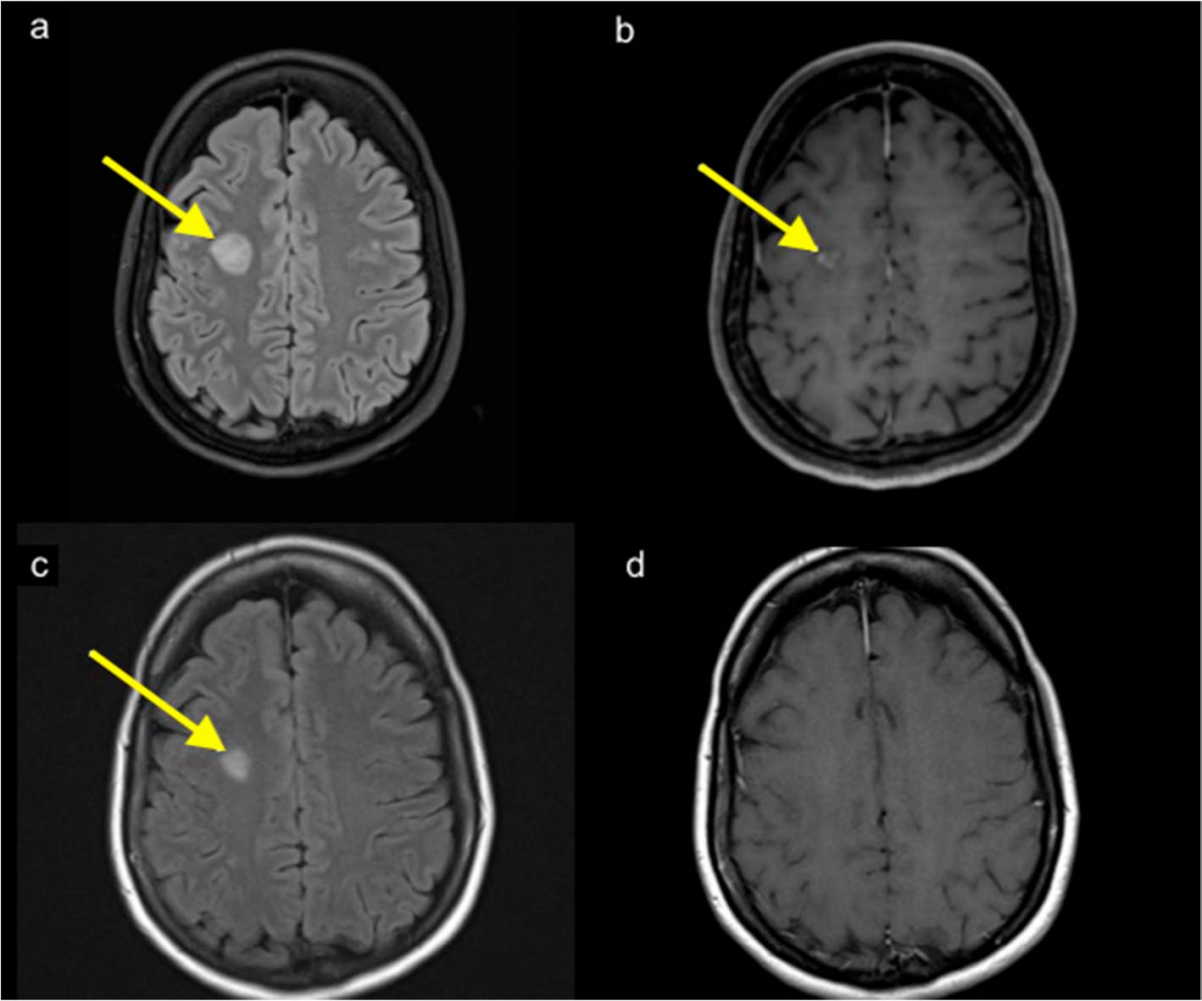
MRI Brain. a. Hyperintense FLAIR signal white matter lesion in the right frontal lobe (arrow). b Internal punctate enhancement of the right frontal lobe lesion (arrow). c Lesion shown in Fig. 1a reduced in size after 2 weeks (arrow). d Lesion no longer demonstrated abnormal enhancement
